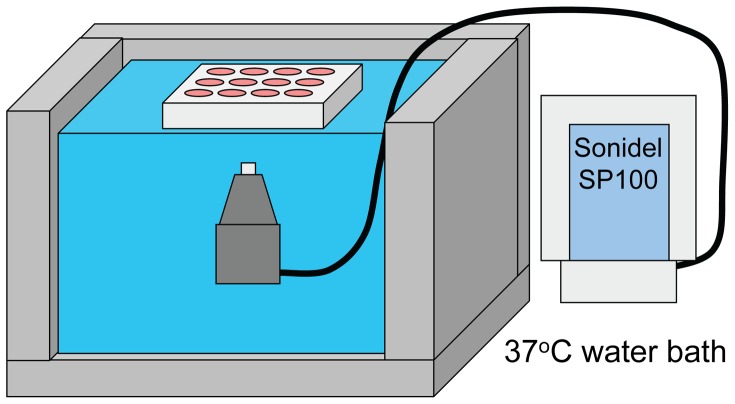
Ultrasound/LSM transfection setup. The bottom of the 12-well plate with cells was submerged in a 37°C water bath. The SP100 ultrasound transducer was placed underneath the plate and ultrasound was emitted upwards into the bottom of each well.
